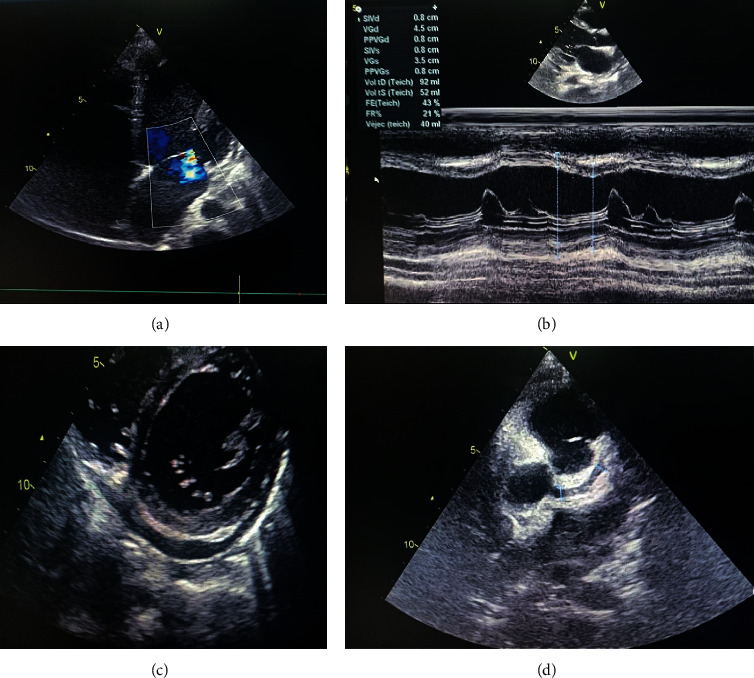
Echocardiographic images showing (a) mitral regurgitation, (b) low left ventricular ejection fraction at 43%, (c) pericardial effusion, and (d) 4 mm coronary dilatation (*Z* score +4).
